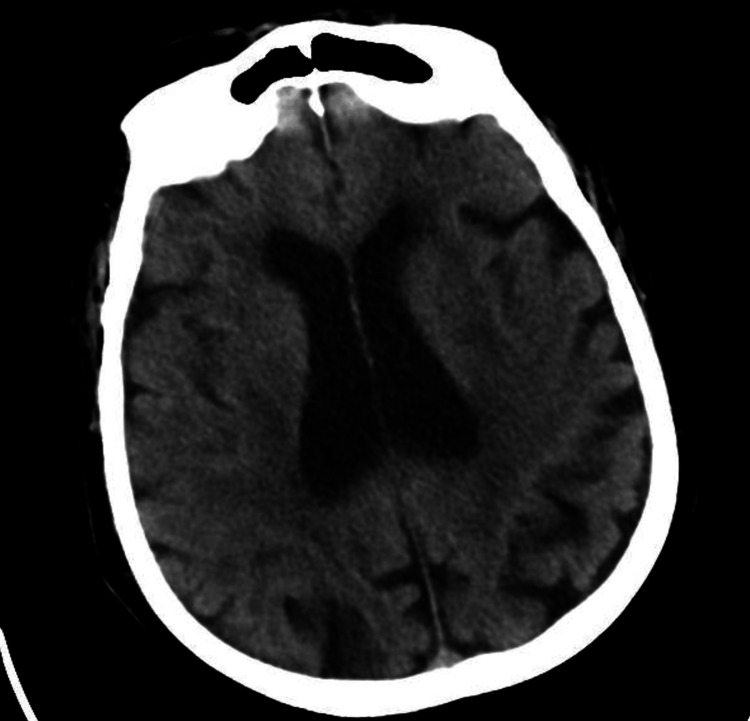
Plain CT scan of the head demonstrating senile atrophy.
